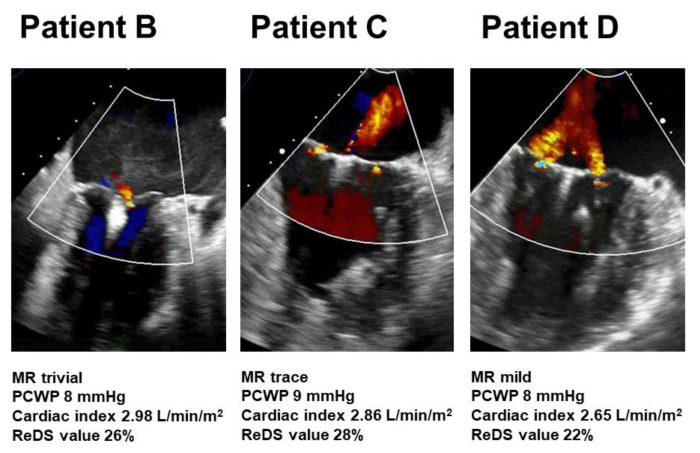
Transesophageal echocardiography following MitraClip procedure in patients B–D. MR, mitral regurgitation; PCWP, pulmonary capillary wedge pressure; ReDS, remote dielectric sensing.
